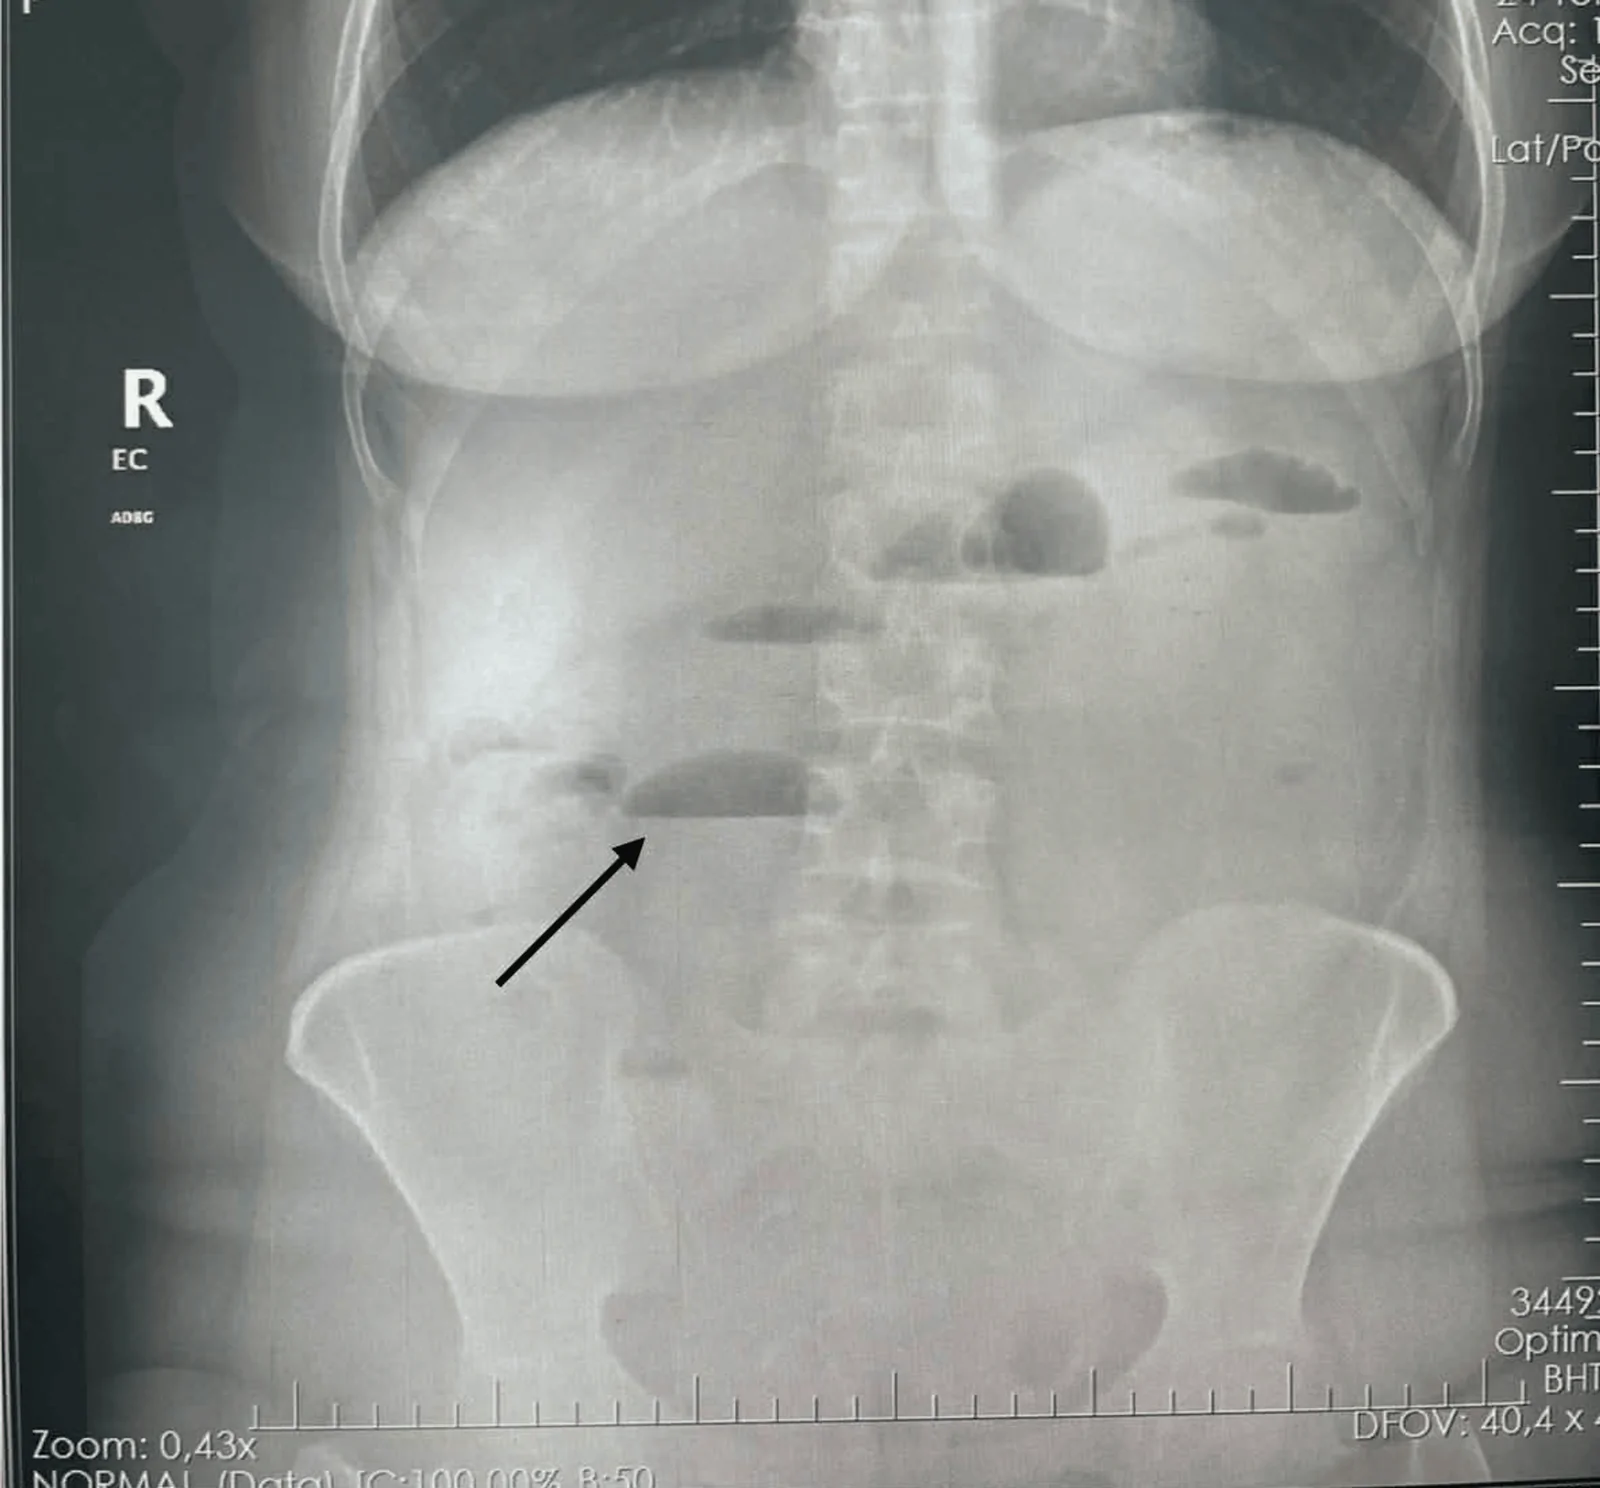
Plain Abdominal Radiograph The image reveals multiple air-fluid levels (arrow), which are classic radiographic findings confirming small bowel obstruction.
